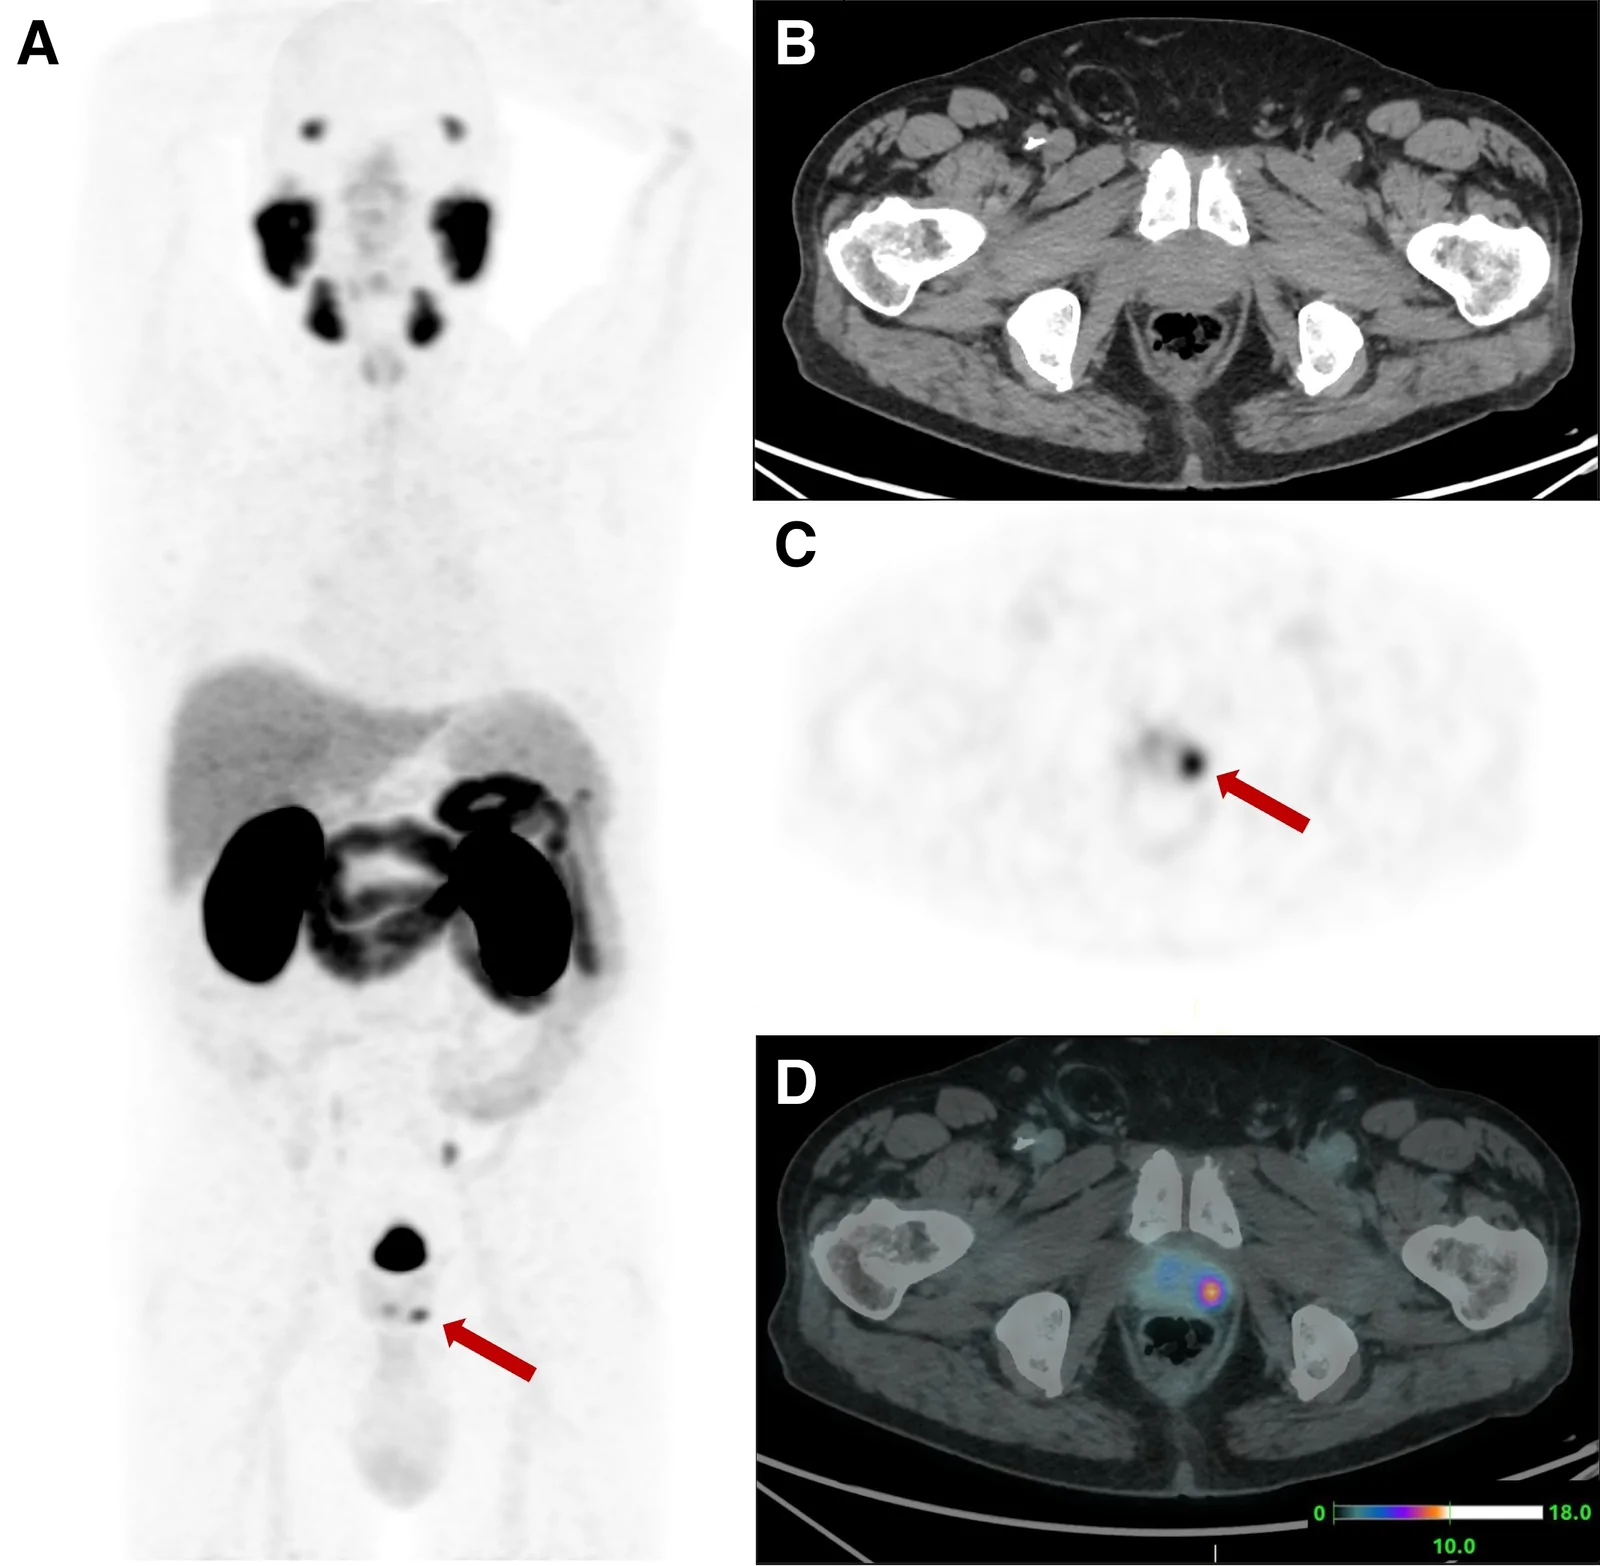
Upstage with PET-guided biopsy. 70-y-old patient with suspicious PCa (PI-RADS 2; initial PSA, 15.9; PSA density, 0.4). Exam showed intense focal [^68^Ga]Ga-PSMA-11 uptake (SUV_max_, 9.5; PRIMARY score 4) in left peripheral zone at midlevel shown maximum-intensity projection (A), transaxial view of CT image (B), PET image (C), and transaxial fused PET/CT image (D). Transperineal PSMA PET Targeted prostate biopsy revealed csPCa, ISUP 5, whereas SB revealed csPCa, ISUP 2.
